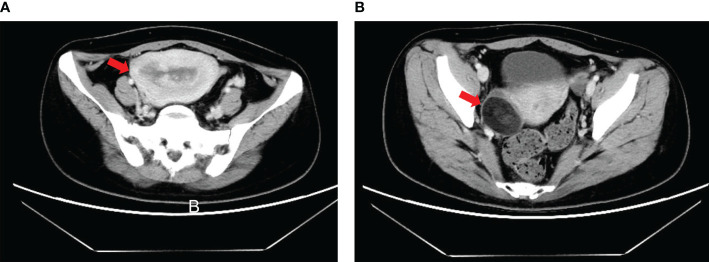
Computed tomography (CT) scan of patient with endometrial YST preoperatively. (A) Enlarged uterus equal to pregnancy for 2 months accompanied by occupancy in the uterus cavity; (B) A 3-cm mass of uneven density in the right adnexa.
